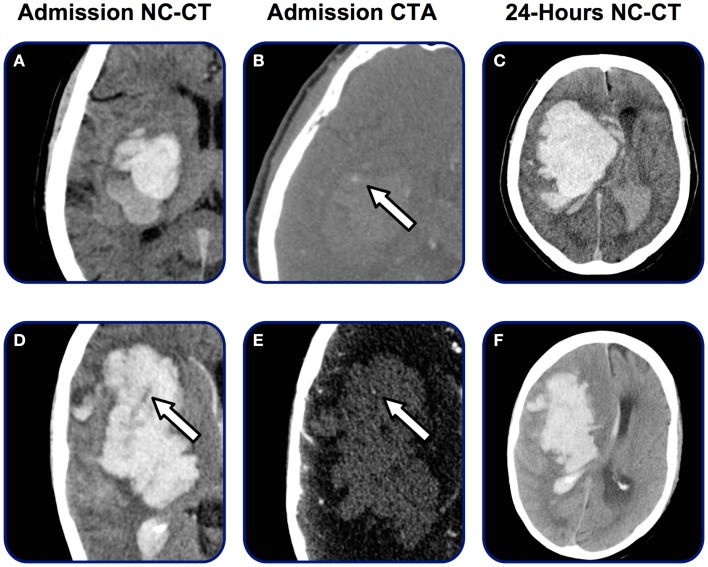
Heterogenicity on admission NC-CT. Admission and follow-up radiology on two patients admitted with acute ICH. NC-CT (A,D) demonstrates marked heterogenic attenuation in hematoma appearance. Arrow on (D) marks swirl-sign. On (B,E) both patients present spot sign. After 24 h (C,F) both patients present enlargement of the hematoma volume and midline shift.
